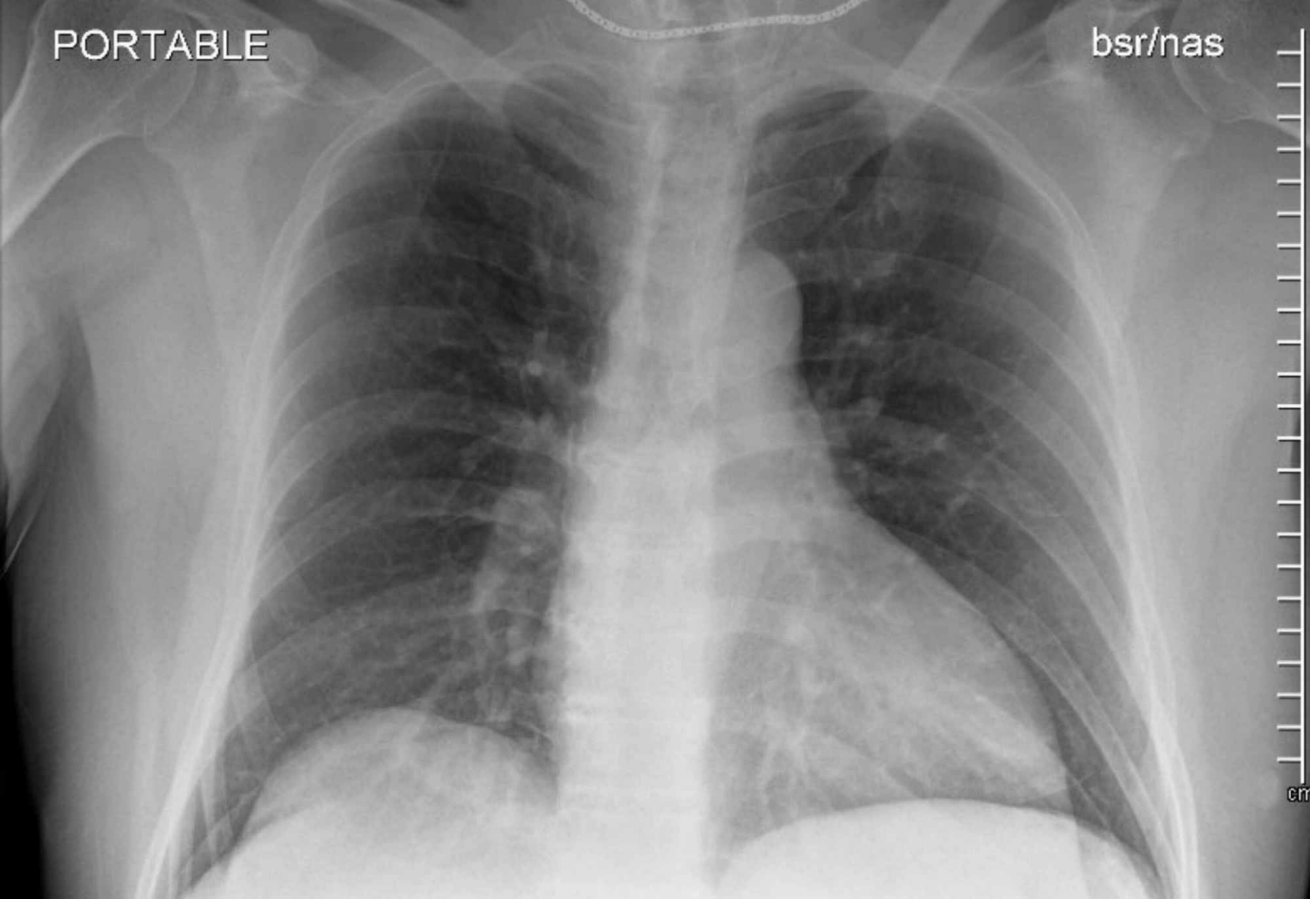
Chest X-ray with no abnormality detected on initial presentation.
